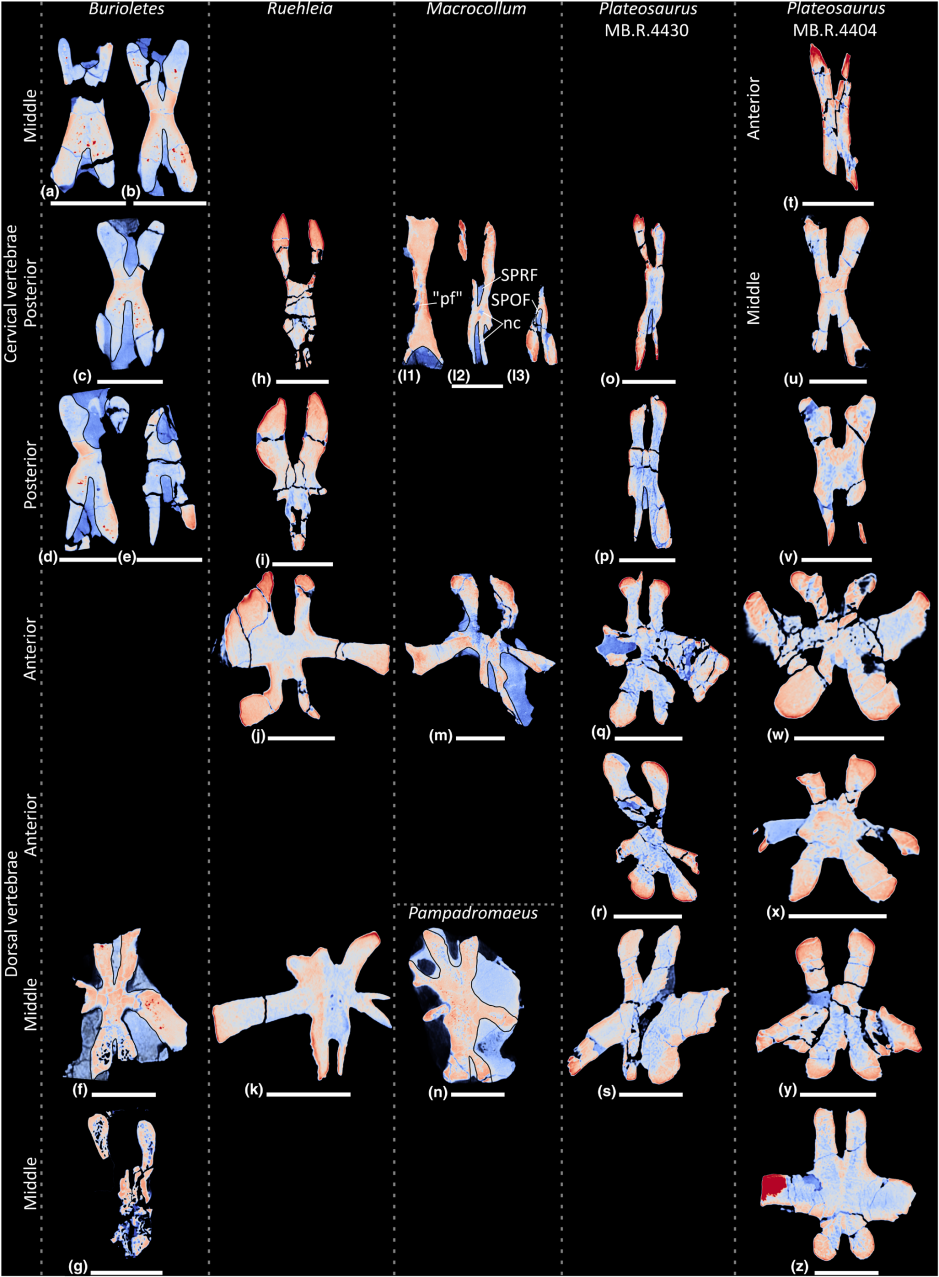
Colour density map outlining PSP in CT scan slices in horizontal cross‐section of the presacral vertebrae of early‐branching sauropodomorphs. (a–g) *Buriolestes schultzi*, (h–k) *Ruehleia bedheimensis*, (l–m) *Macrocollum itaquii*, (n) *Pampadromaeus barberenai*, (o–z) *Plateosaurus longiceps*; (t) anterior cervical vertebra, (a–b, u) middle cervical vertebrae, (c–e, h–i, l, o–p, v) posterior cervical vertebrae, (j, m, q–r, w–x) anterior dorsal vertebrae, (f–g, k, n, s, y–z) middle dorsal vertebrae; (l1) CT scan slice through centrum, (l2) CT scan slice through neural arch, (l3) CT scan slice through neural spine. nc, neural canal; ‘pf’, pneumatic foramen; SPOF, spinopostzygapophyseal fossa; SPRF, spinoprezygapophyseal fossa. Scale bars = 2 cm (*Buriolestes*, *Macrocollum*, *Pampadromaeus*), 5 cm (*Ruehleia*, *Plateosaurus*).
